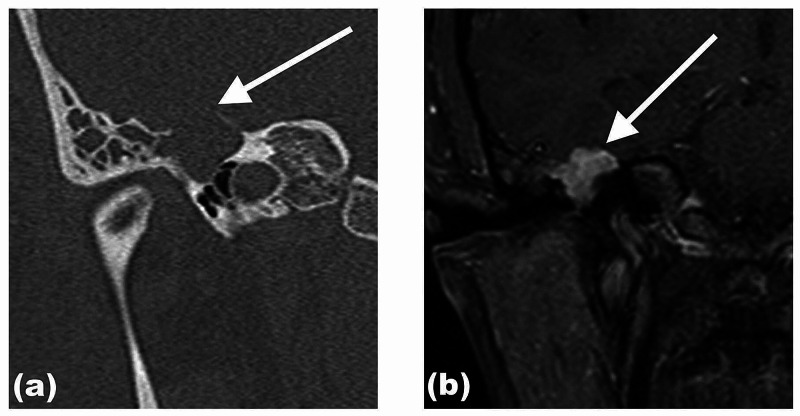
Coronal reformatted view of HRCT of the right temporal bone (a) and contrast-enhanced T1-weighted image (b) Coronal reformatted view of HRCT of the right temporal bone (a) and contrast-enhanced T1-weighted image (b). The facial nerve schwannoma is causing a widening of the epitympanic space (a, white arrow) and extending into the mastoid air cells. On MRI, there is enhancement seen of the facial nerve schwannoma and extension to the middle cranial fossa as extradural mass (b, white arrow). HRCT - high-resolution computed tomography
